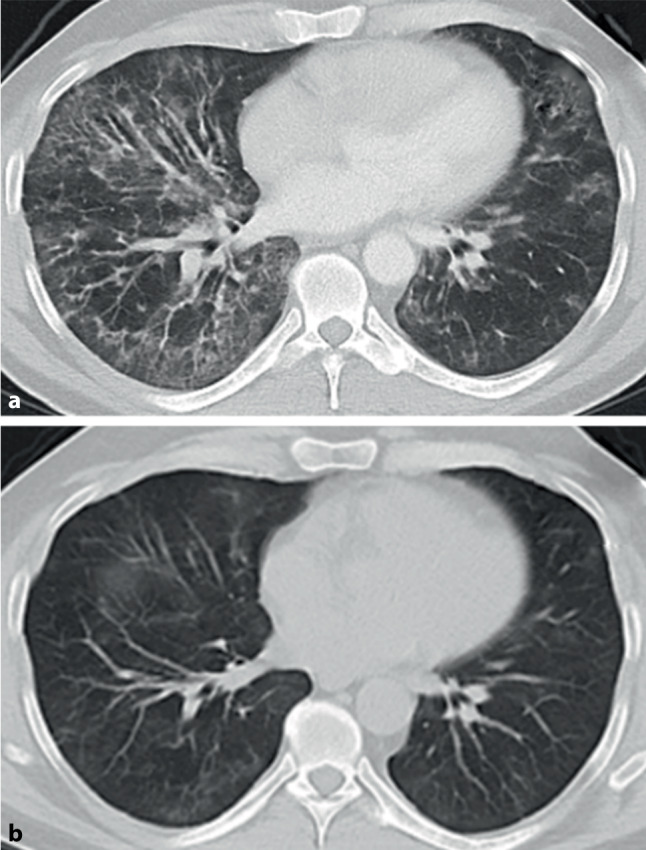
Die HRCT kann zur Verlaufsbeurteilung bei pfILD herangezogen werden [27], allerdings sollten immer auch andere Verlaufsparameter, z. B. die Lungenfunktion einschließlich der Diffusionskapazität und die Symptomlast, berücksichtigt werden, da die Vergleichbarkeit von HRCT-Schnitten auch durch technische oder patientenseitige Faktoren eingeschränkt sein kann. Zukünftig könnten hier computerbasierte quantitative Auswertungsalgorithmen zum Einsatz kommen, die derzeit in der klinischen Routine aber noch nicht verfügbar sind [27, 33].
